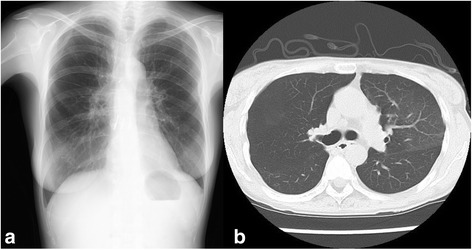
Chest radiography (a) and computed tomography (b) images of the patient before minimally invasive esophagectomy. a Normalized lung fields, and sternal wires and marking clips on mediastinal field are seen. b Normalized lung fields and marking clips on the esophagus are seen
